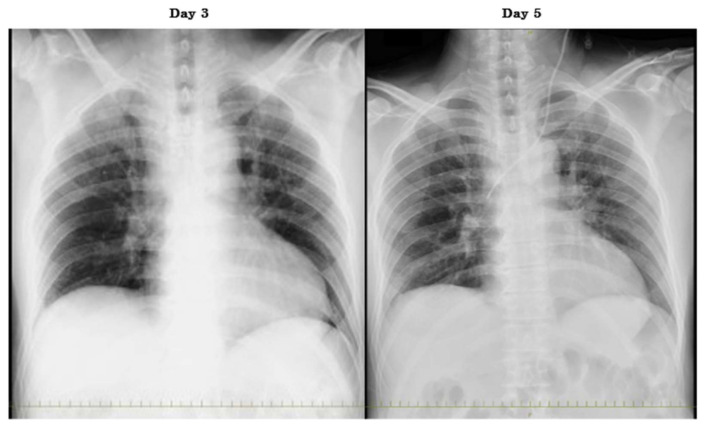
Chest radiograph. Compared to day 3, an air bronchogram appeared at the upper- and middle-lung fields on the left side on day 5.
